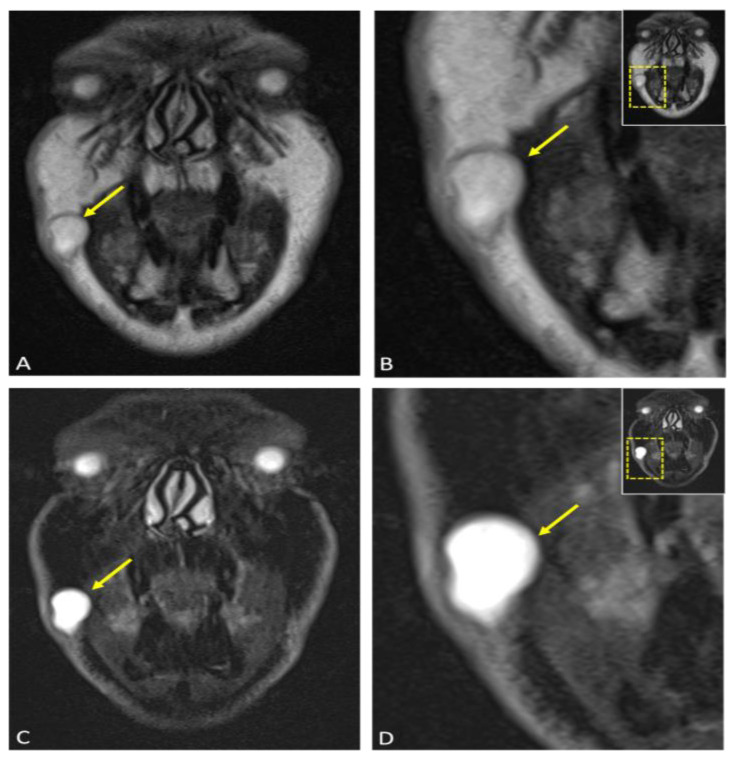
Coronal reconstructions of the lesion (arrow) are displayed in (A) T2-weighted Dixon MRI in-phase (water + fat) and (C) T2-weighted Dixon MRI water-only phase, which can be used as a fat-suppressed image. Enlarged views of the lesion are provided in (B,D) for greater clarity. The imaging findings are consistent with an epidermoid cyst, characterized by a well-circumscribed appearance and lack of infiltration into adjacent structures or inflammatory changes.
